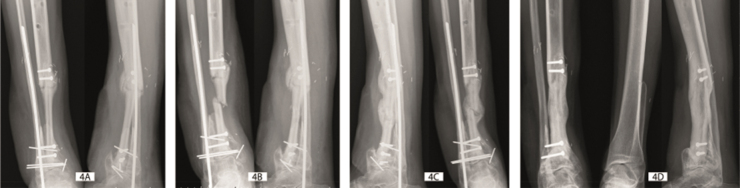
A. Reconstruction of distal tibia after a crush injury due to motorcycle accident. X-rays taken during the 4^th^ postoperative month reveal a bony union of the flap host interface. The patient wears a total contact brace to protect the slender fibular flap. B. X-rays taken during the 5^th^ postoperative month reveal a stress fracture of the flap. C. X-rays taken during the 7^th^ postoperative month depict a robust callus formation at the fracture site, triggering new periosteal bone formation throughout the fibular flap. D. X-rays taken during the 36^th^ postoperative month reveal massive bone hypertrophy, where the girth of the flap is slightly wider than that of the reconstructed bone.
